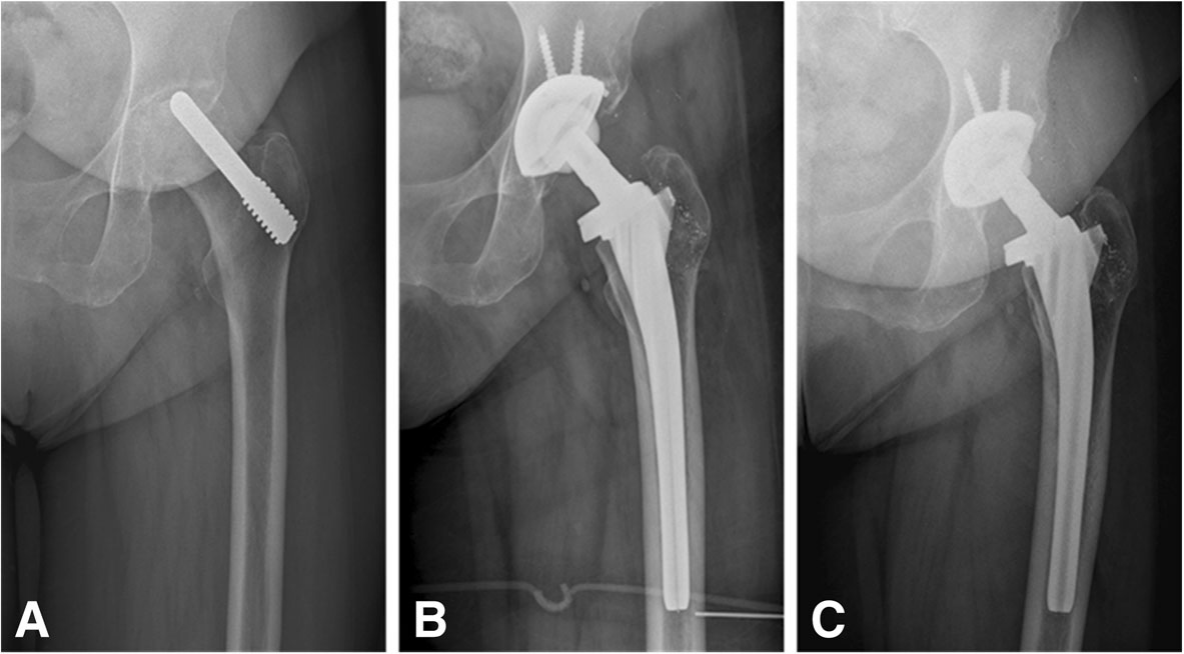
Radiographs of the left hip of a 51-year-old woman with ONFH after insertion of the tantalum implant with later conversion to total hip replacement with a ceramic-on-polyethylene bearing. a Pre-operative radiograph. b Anteroposterior postoperative radiograph at 1-year follow-up shows no osteolysis or radiolucencies. Tantalum debris can be observed in the femoral proximal region. c Radiograph at the 7-year follow-up shows no changes
